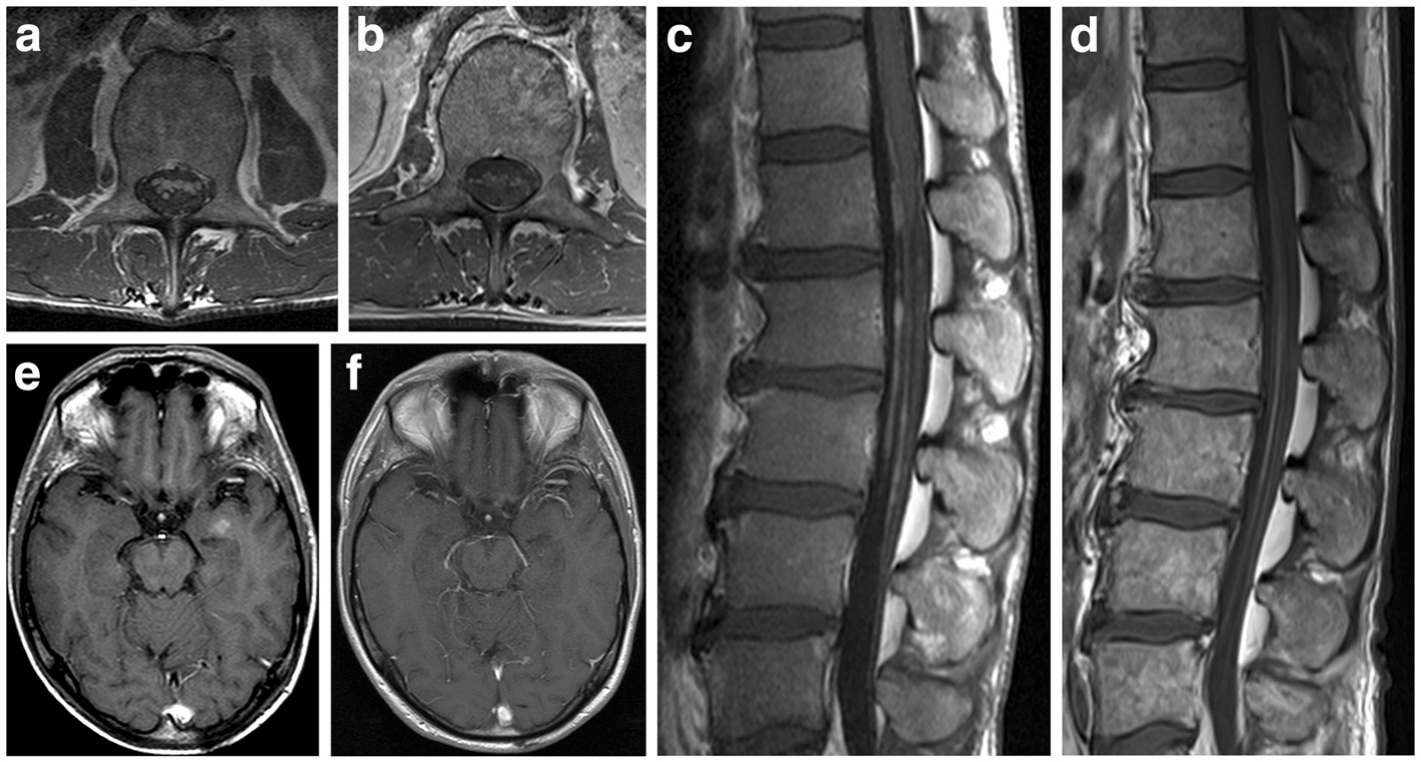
MR images of LWS (a-d) and cranium (e-f) at initial diagnosis (a, c, e) and at 24 months after allogeneic transplantation (b, d, f). The size and the intensity of contrast-agent enhanced structures within CNS have regressed after stem cell transplantation.
